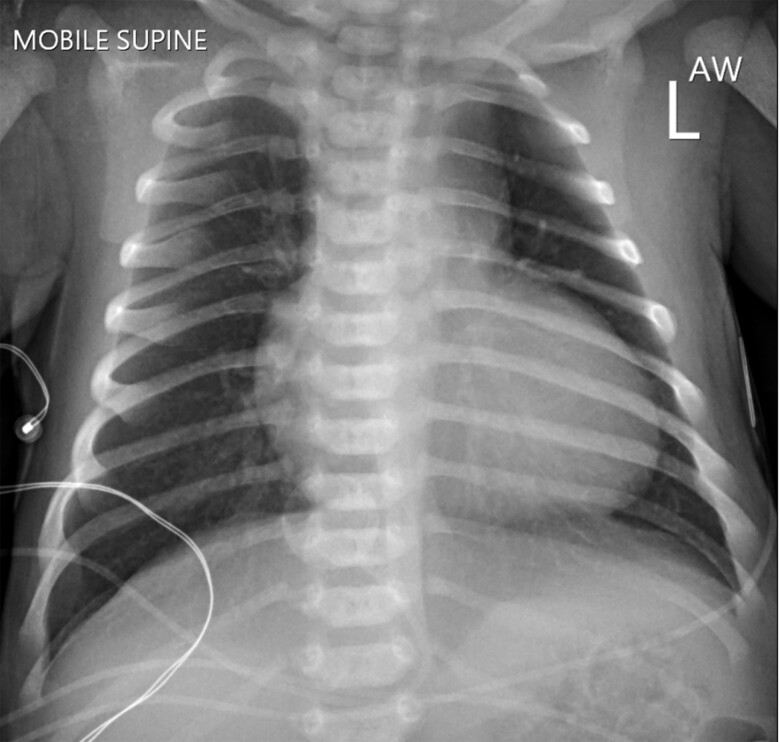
Chest x-ray on day 2 of life demonstrating mild cardiomegaly and no other abnormalities.
